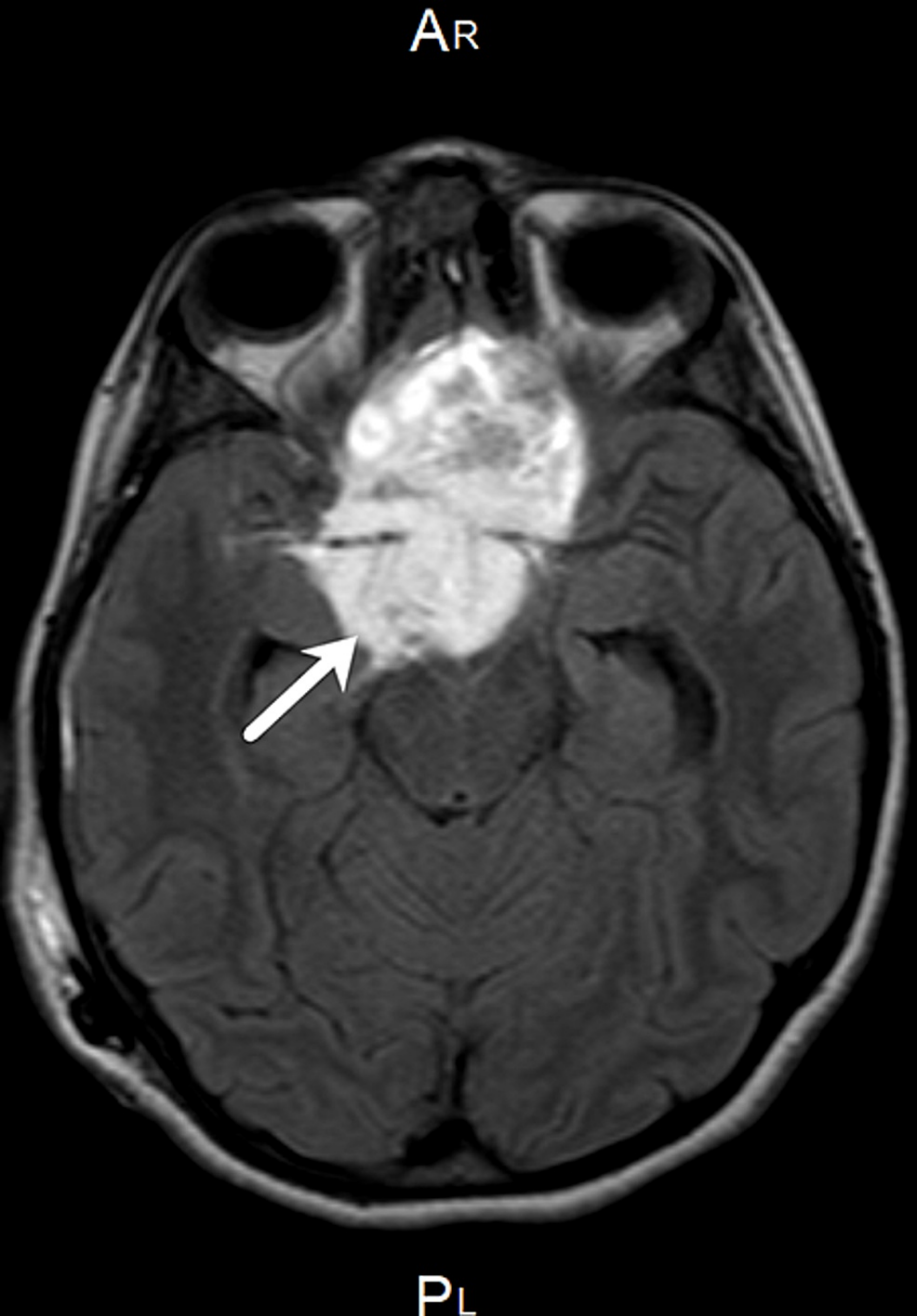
T1-weighted brain MRI post-contrast axial view shows a large sellar/supraseller mass engulfing the anterior aspect of the circle of the Willis and extending to the third ventricle.
